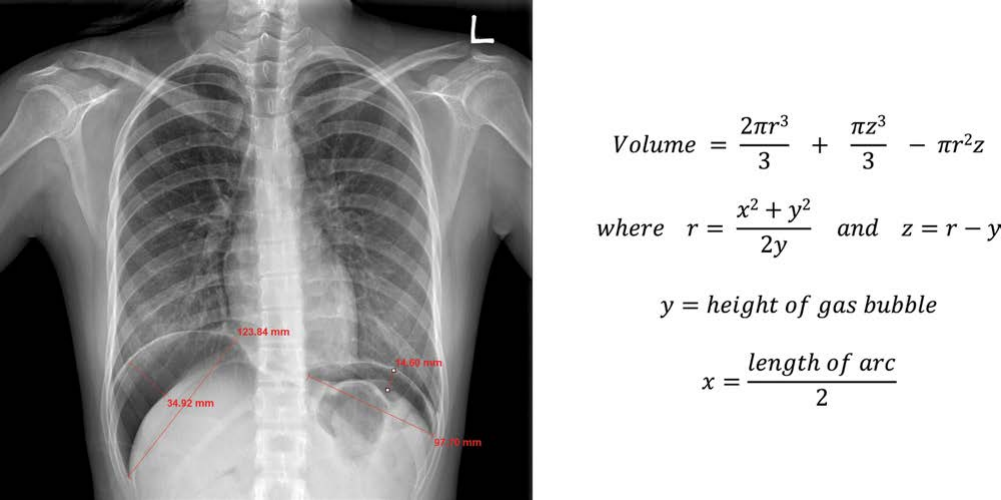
Chest X-ray (left) showing large gas bubbles under each hemidiaphragm. Dimensions on the right: length of arc, 12.4 cm; height, 3.5 cm; volume, 233.7 mL. Dimensions on the left: length of arc, 9.8 cm; height, 1.5 cm; volume, 58.3 mL. All volumes were calculated using the formulas shown on the right.
